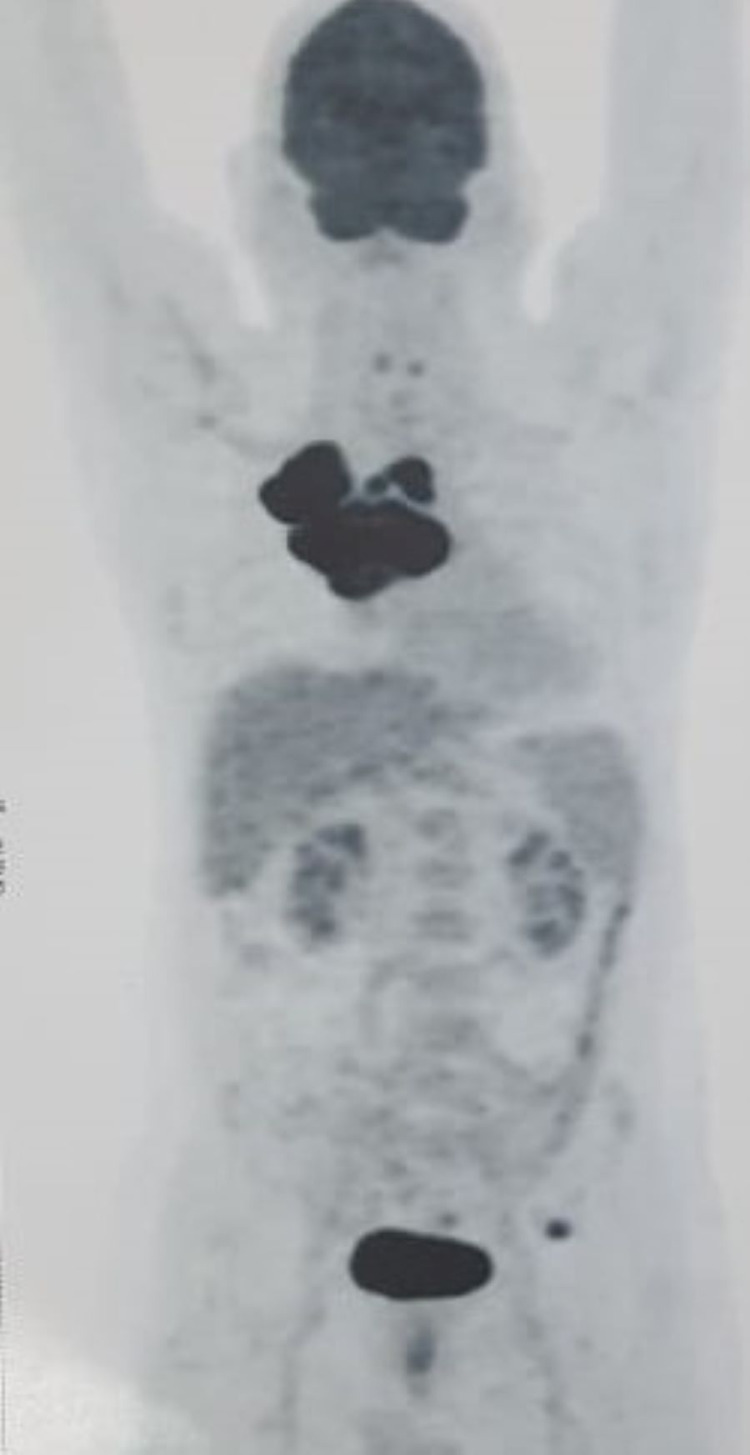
Whole-body PET-CT scan showing significant interval increase in metabolic activity at primary site. PET-CT, positron emission tomography-computed tomography.
